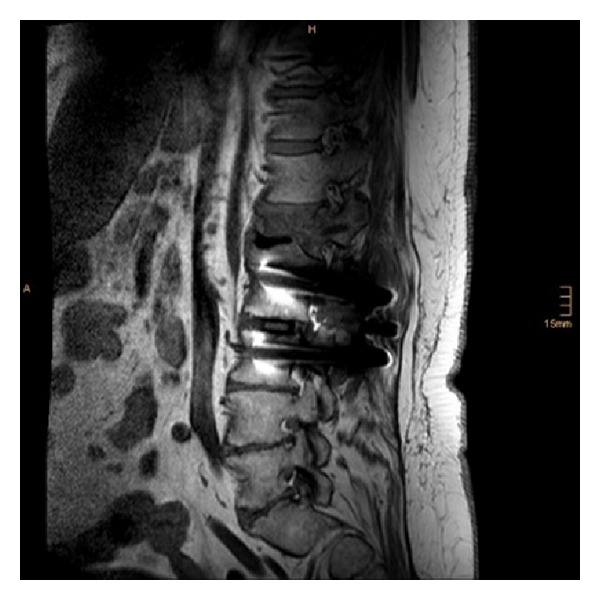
Postoperative sagittal MRI (T1-weighted) at the level of the pedicles (right).
